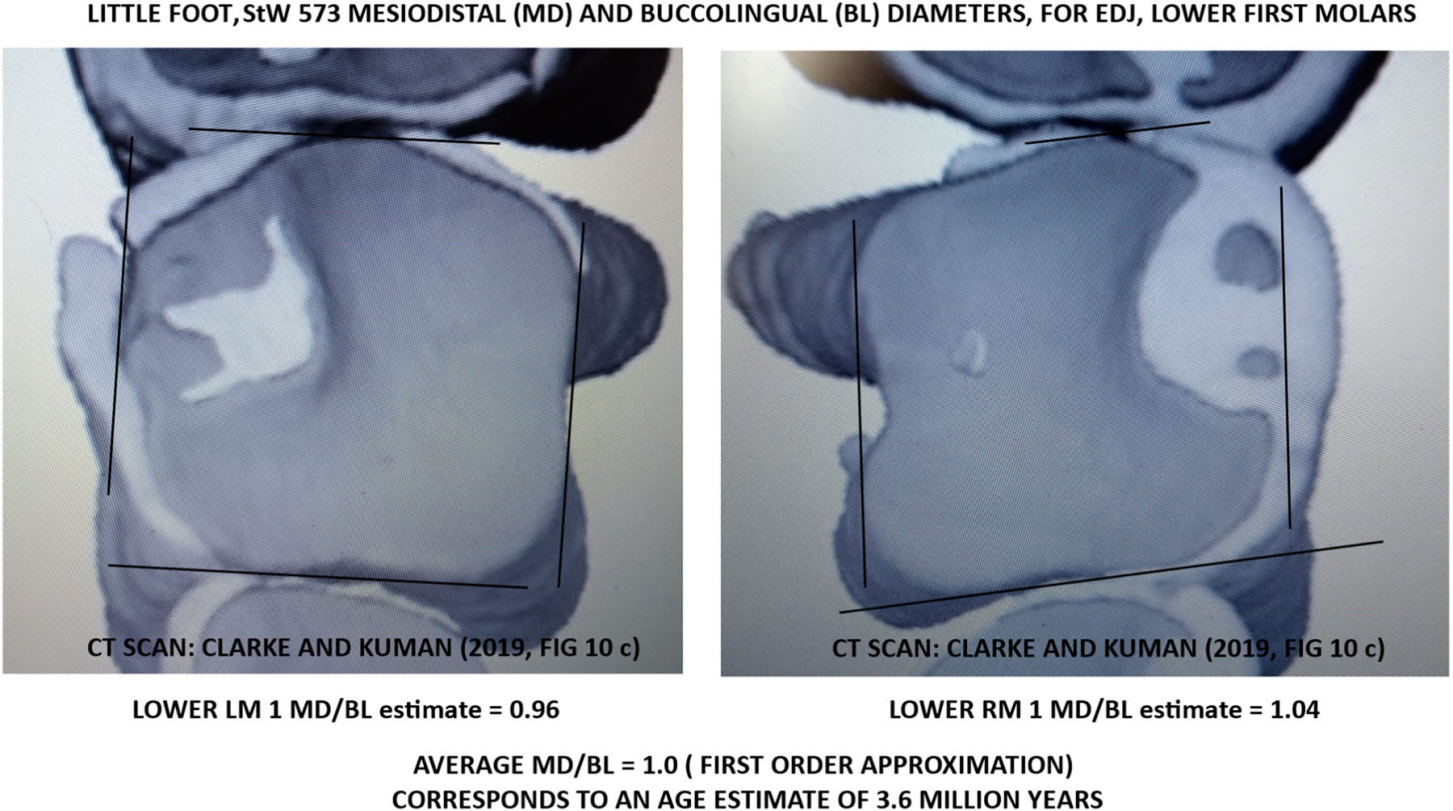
CT scans of left and right lower first molars of StW 573 (from Clarke and Kuman,^12^ their fig. 10c), indicating reference lines for MD and BL dimensions estimated from the enamel‐dentine junction (EDJ). BL, buccolingual; CT, computed tomography; MD, mesiodistal.
